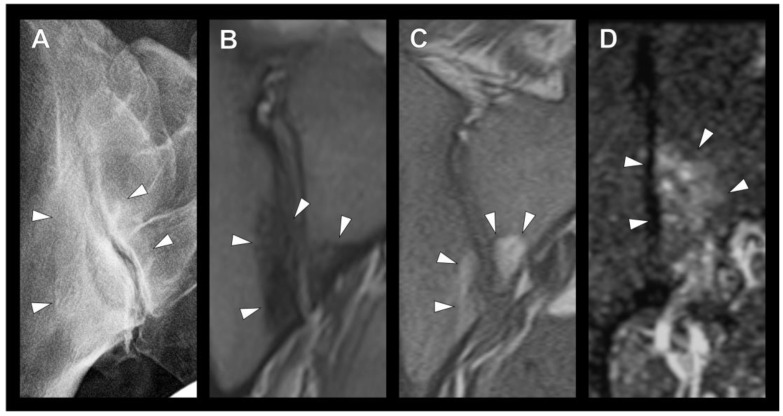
Arrowheads indicate the detected lesion. (A) Radiograph of a right sacroiliac joint (SIJ) with osteitis condensans ilii. (B–D) MR images of the SIJ with the presence of (B) sclerosis, (C) fatty bone marrow metaplasia and (D) bone marrow edema in the typical site of OCI.
